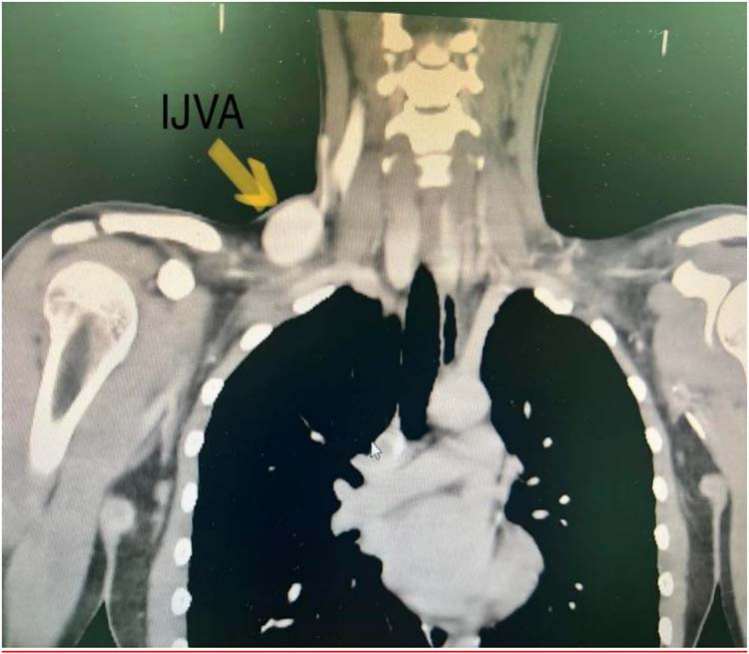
CT with IV contrast showing IJV aneurysm. CT with intravenous contrast showed aneurysmal fusiform venous tributary in right-sided lower neck that originate from right IJV and rejoins IJV before its insertion with the right subclavian vein to form the innominate vein measuring 6 cm in length and 4 cm in maximum diameter. There was no compressing mass in the chest or neck. SVC was normal with no signs of stenosis or compression.
